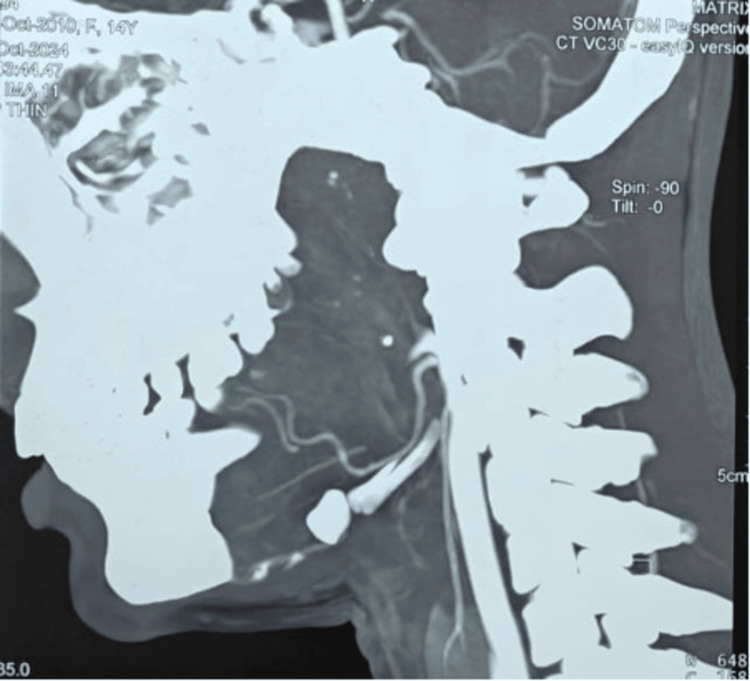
CT angiogram showing facial artery as feeder vessel. Slice thickness: 1 mm at 1 mm interval.
